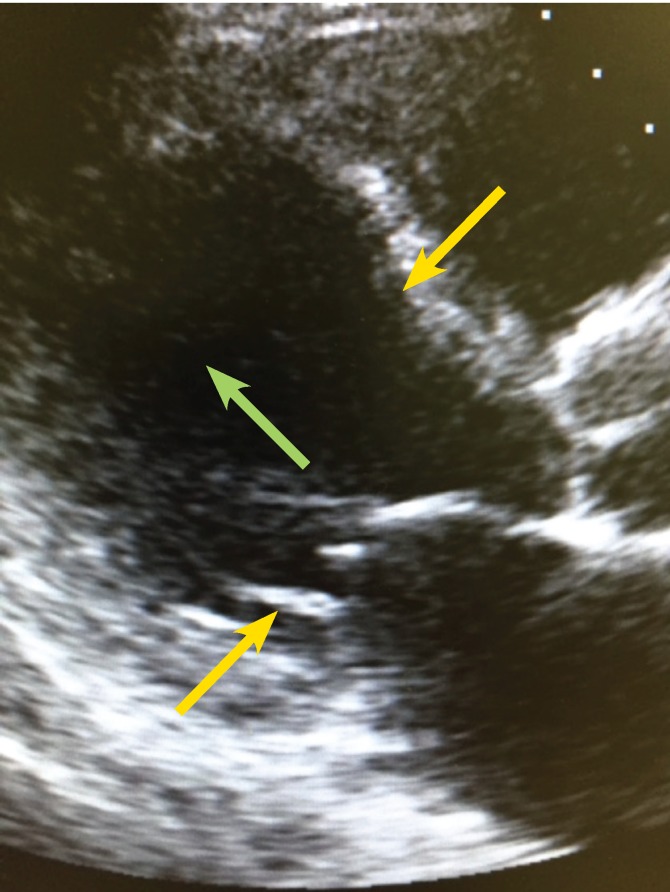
Parasternal long-axis view showing apical and midcavity ballooning (green arrow) and basal hypercontractility (yellow arrows).
